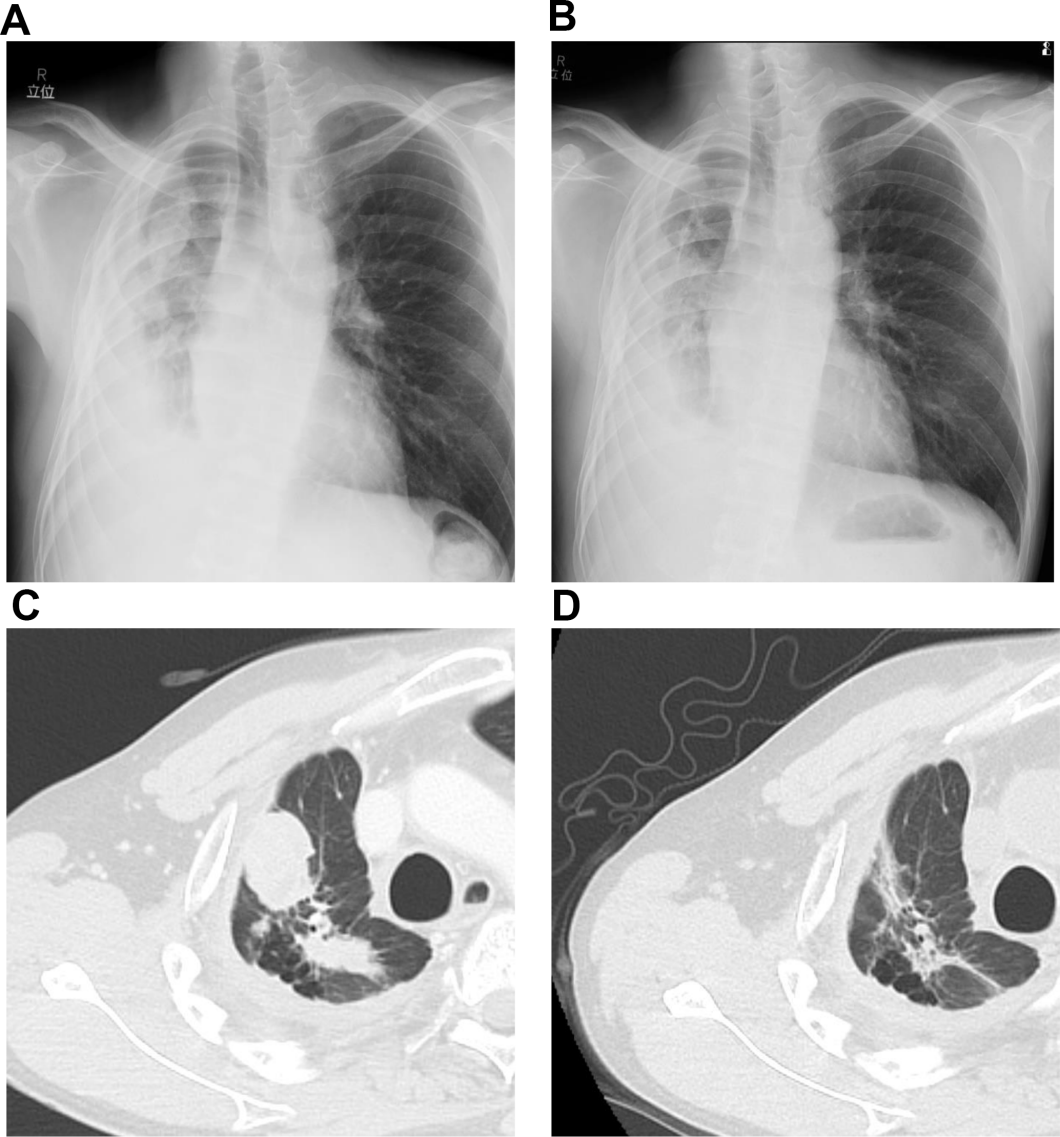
(A, C) Chest radiograph and computed tomography scan before starting ceritinib. (B, D) Chest radiograph and computed tomography scan after ceritinib treatment.
